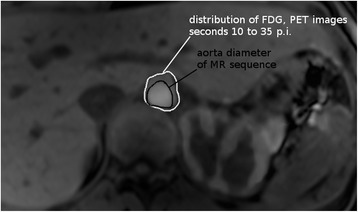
Fused image of MRI T1 sequence and PET. From the latter, the sum of the second to seventh frame (i.e., seconds 10 to 35) p.i. is shown. Due to partial volume effects, the FDG distribution in the PET images (white contour) clearly exceeds the aorta diameter observed with MRI image (black contour). Also, the initial accumulation of FDG in the cortex is visible
